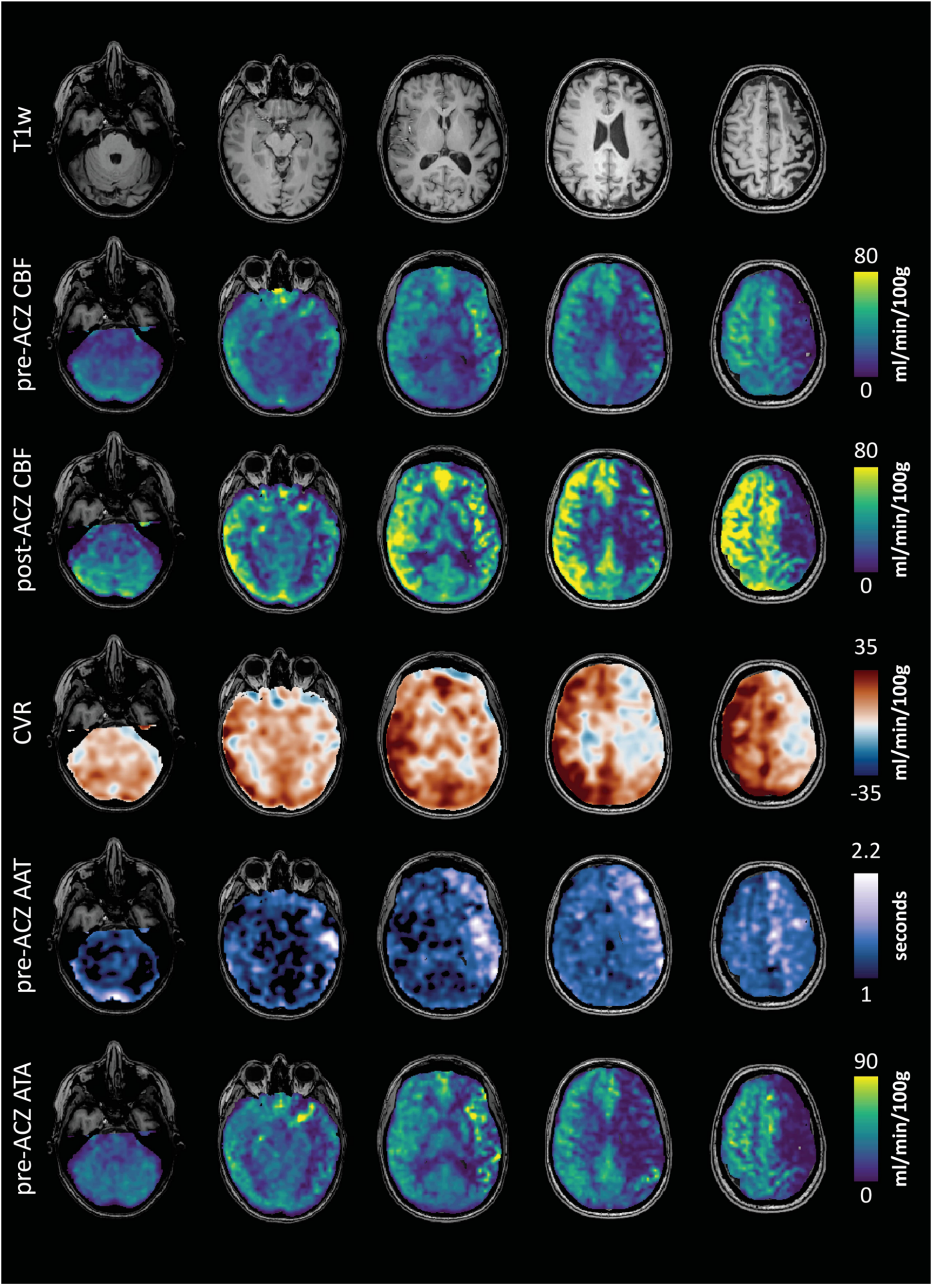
Hemodynamic maps derived from ASL, including pre-acetazolamide CBF, post-acetazolamide CBF, CVR, pre-acetazolamide arterial arrival time (AAT), and pre-acetazolamide arterial transit artifacts (ATAs). In clinical practice, each map consists of sixteen (caudal to cranial) axial ASL-MRI slices (see Fig. S3). The pre-acetazolamide CBF maps show a lower CBF in the left MCA territory, with prolonged AAT, as well as multiple ATAs in the corresponding territory. The post-CBF map shows a normal increase in CBF in the cerebellum and right hemisphere, but no response in the left hemisphere. Hence, the CVR map (calculated by subtracting the pre-acetazolamide CBF map from the post-acetazolamide CBF map) indicates a large are of steal in the left hemisphere. This is caused by a severe stenosis of the left M1 on MRA (not shown)
